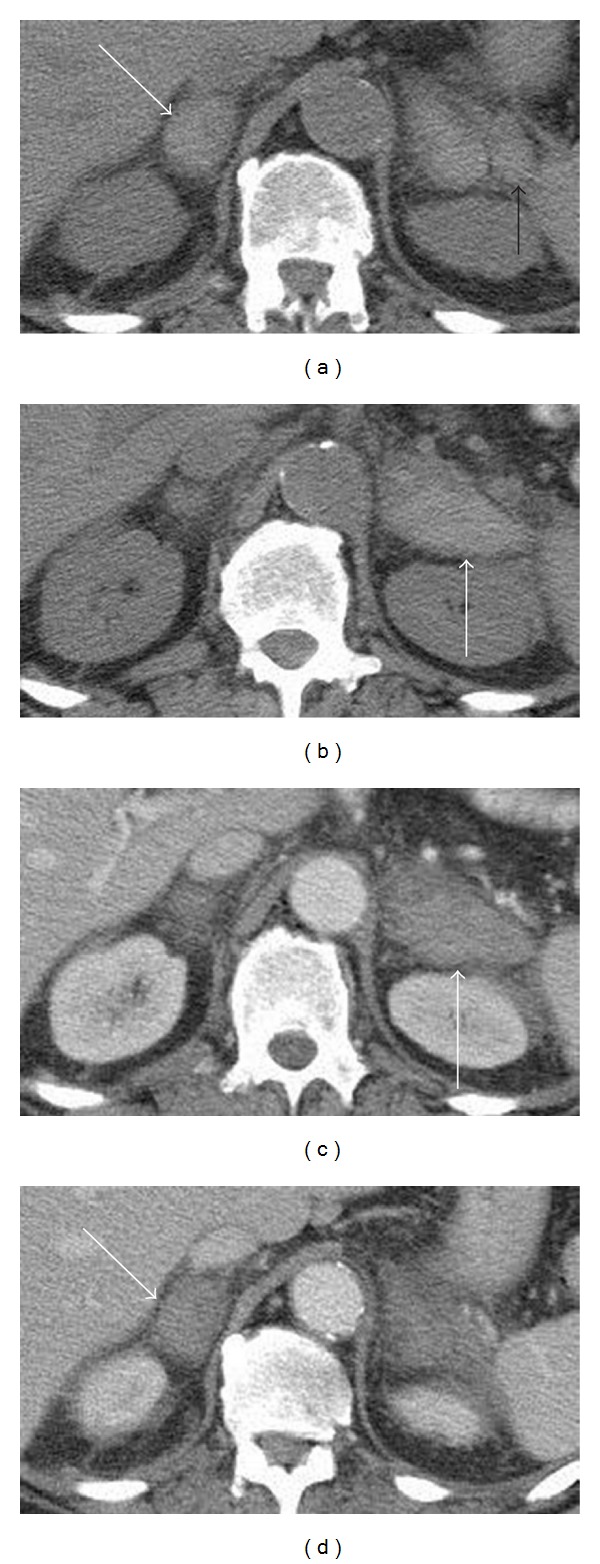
Axial non-contrast-enhanced CT image (a) shows bilateral adrenal enlargement (white arrows) with high attenuation values. Axial contrast-enhanced CT image (b) shows a bilateral homogeneously enhancing adrenal mass with solid appearance. Note the stranding of the periadrenal fat (black arrow) and the lack of regional lymphadenopathy.
